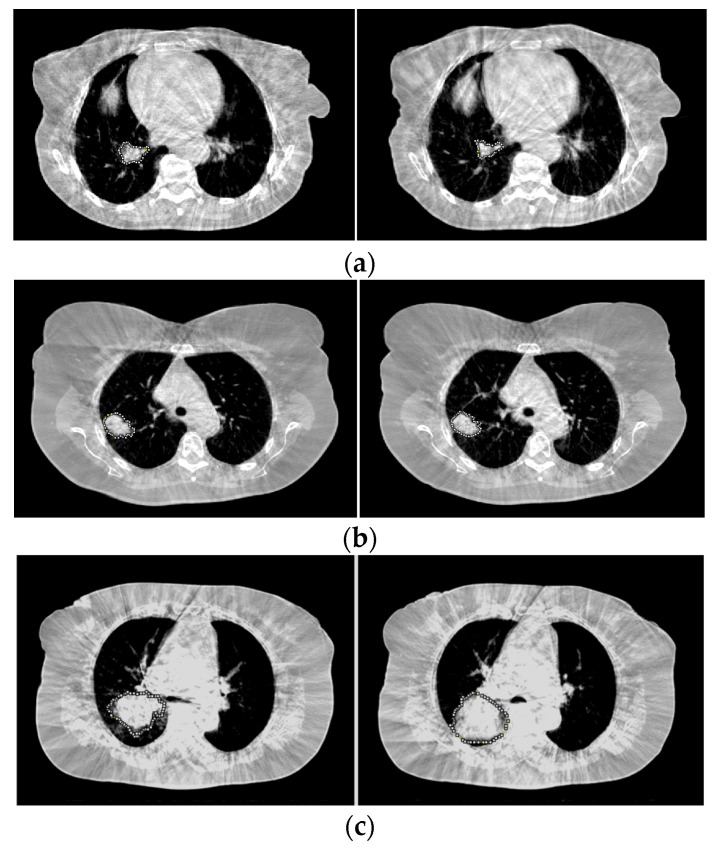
Tumor change between CBCT 1 (left) and CBCT 5 (right). *R_A_*, *R_CNR_*, *R_μ_*, and *R* are consistent with RA. (a) Response; (b) stable; (c) progression. Tumors are contoured by an iso-pixel line.
